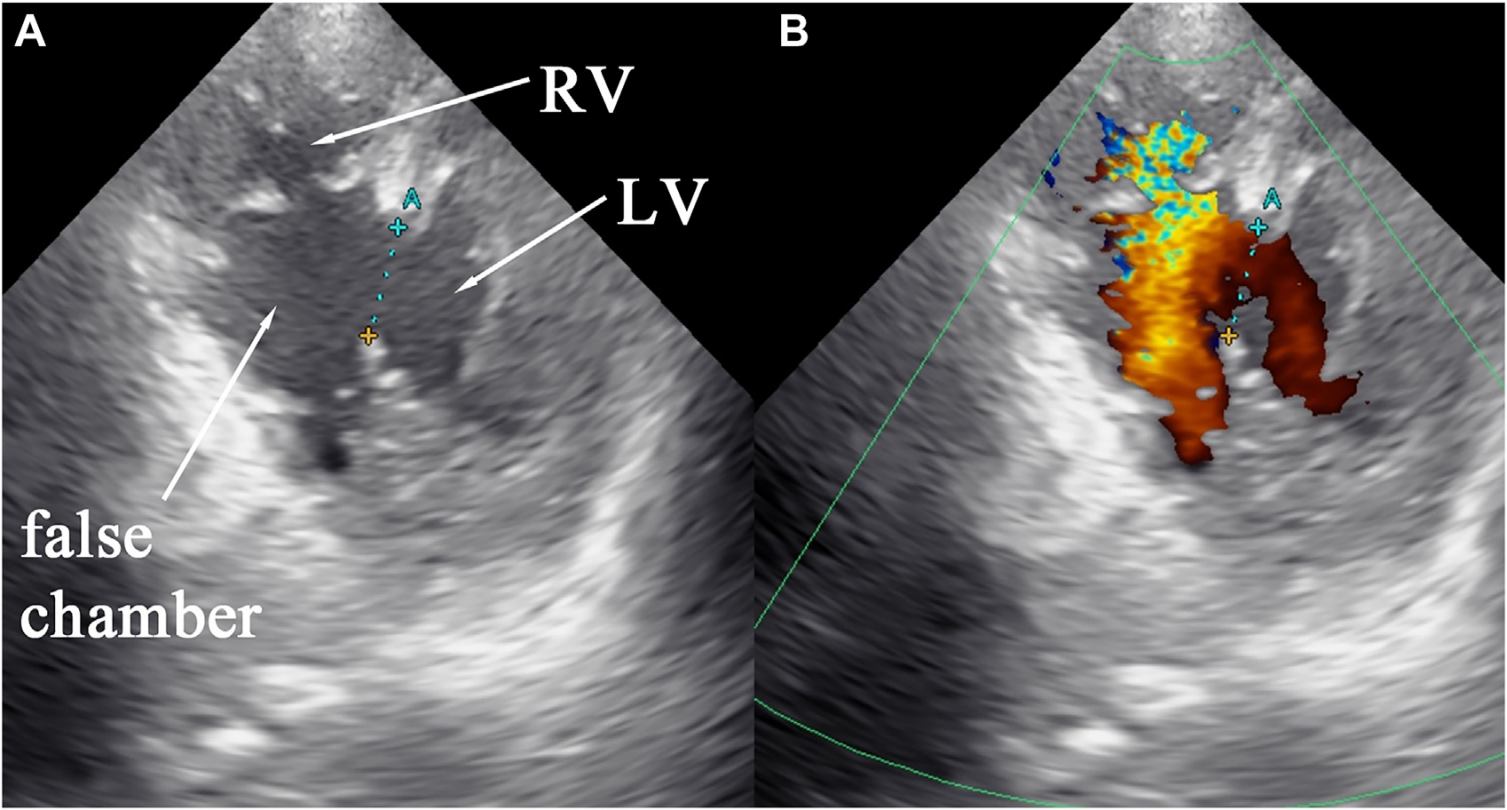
Short-axis transthoracic echocardiography. (A) Echocardiography shows the right ventricle (RV), the left ventricle (LV), the false chamber, and an 18-mm defect in the posterior ventricular septum. (B) Ventricular septal rupture continuous with the large dissected space is also continuous with the true lumen of the right ventricle.
